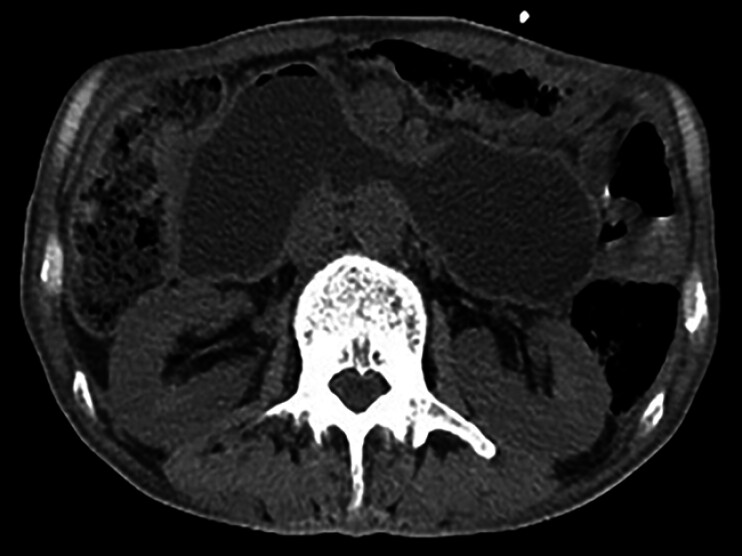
Computed tomography showed duodenal dilation and fluid accumulation.
